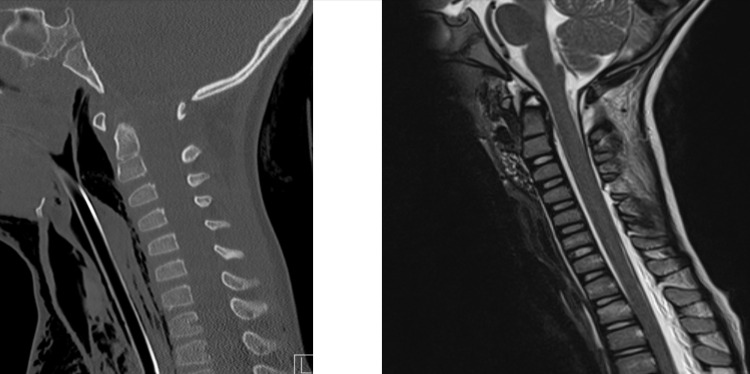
Preoperative CT (left) and MRI (right) scan CT imaging demonstrates atlantoaxial instability and widening of the C1-2 articular space to 6 mm and an ADI of 5 mm. The Powers ratio was normal. Notably, there was extensive soft tissue emphysema in the neck. MRI imaging revealed a partial tear in the right alar ligament. ADI: atlantodens interval
